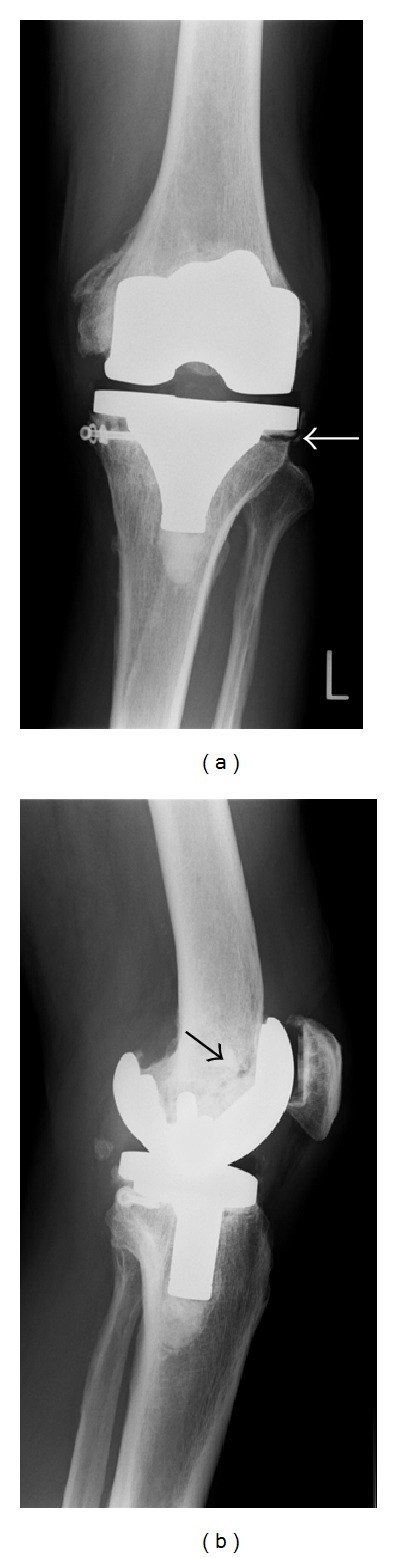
Radiographs of the left knee immediately after the patient sprained his knee and became unable to walk. An anteroposterior radiograph shows a radiolucency 1 mm in depth beneath the lateral aspect of the tibial component ((a), arrow), and the lateral view indicates a condylar fracture ((b), arrow).
